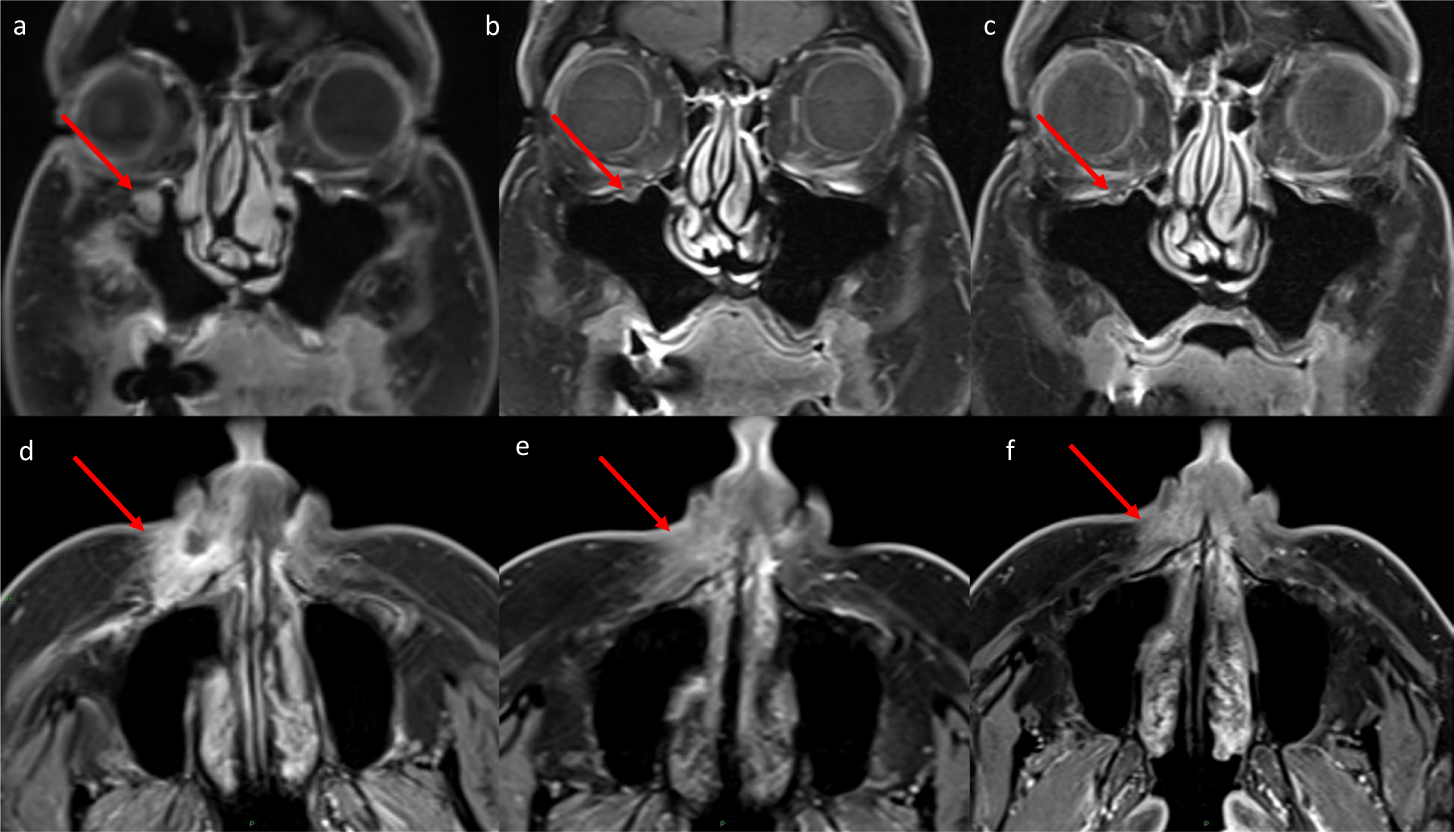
Magnetic resonance imaging (MRI) demonstrating tumour RECIST 1.1 response with concordant perineural spread (PNS) response: (A) All images MRI post-contrast T1 fat saturation (FS). Coronal MRI images demonstrate: (A) Baseline. Arrow indicates a thickened and enhancing right maxillary division trigeminal nerve (V2). B < 5 months image: diminished size and enhancement of the right maxillary division trigeminal nerve. C > 10 months image: no enhancement of the right maxillary division trigeminal nerve with normal size. Axial MRI images demonstrate: (D) Baseline. Enhancing left malar mass cutaneous squamous cell carcinoma (CSCC). E < 5 months, diminished enhancement and mass. F > 10 months (28 months), resolved enhancement and mass
